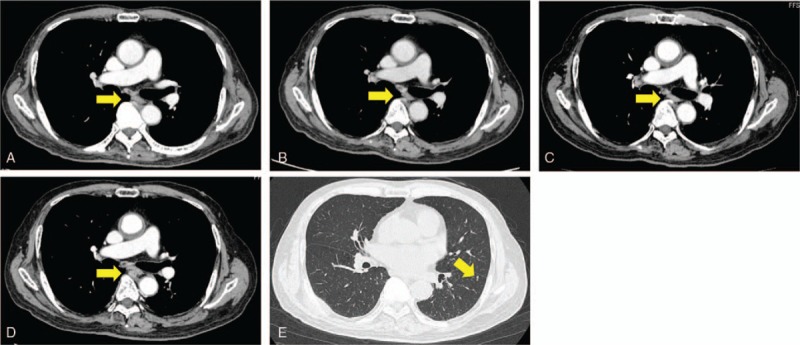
CT of the thorax after dosing with trabectedin. (A) April 2017. (B) November 2017. (C) May 2018. (D) September 2018 after 11 cycles of trabectedin. No significant change was observed in the lymph node metastasis around the artery (arrow). (E) CT showing new lung metastasis at the left inferior lobe (arrow). CT = computed tomography.
